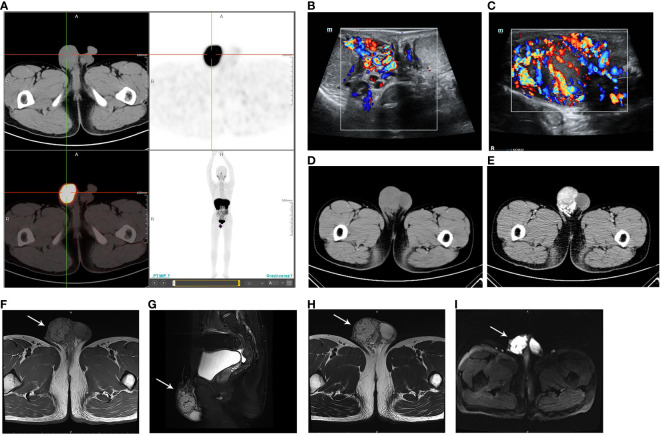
(A) Ga^68^ DOTATATE PET-CT showing a mass in the right scrotum, with a SUVmax value of 26.7 and a size of 38 × 47 mm. The DOTATATE uptake of the mass is significantly increased. (B) Scrotum ultrasound confirming a mass in the right tunica vaginalis cavity (C) with abundant blood flow signals. (D) Testicular CT demonstrating a low-density mass in the right scrotum. (E) The mass is obviously enhanced in contrast-enhanced imaging. (F) MRI revealing the low T1-weighted signal of the lesion. (G) Sagittal plane and (H) transverse section MRI images showing the high T2-weighted signal of the lesion. (I) Lesion presenting as hyperintense on diffusion-weighted imaging.
